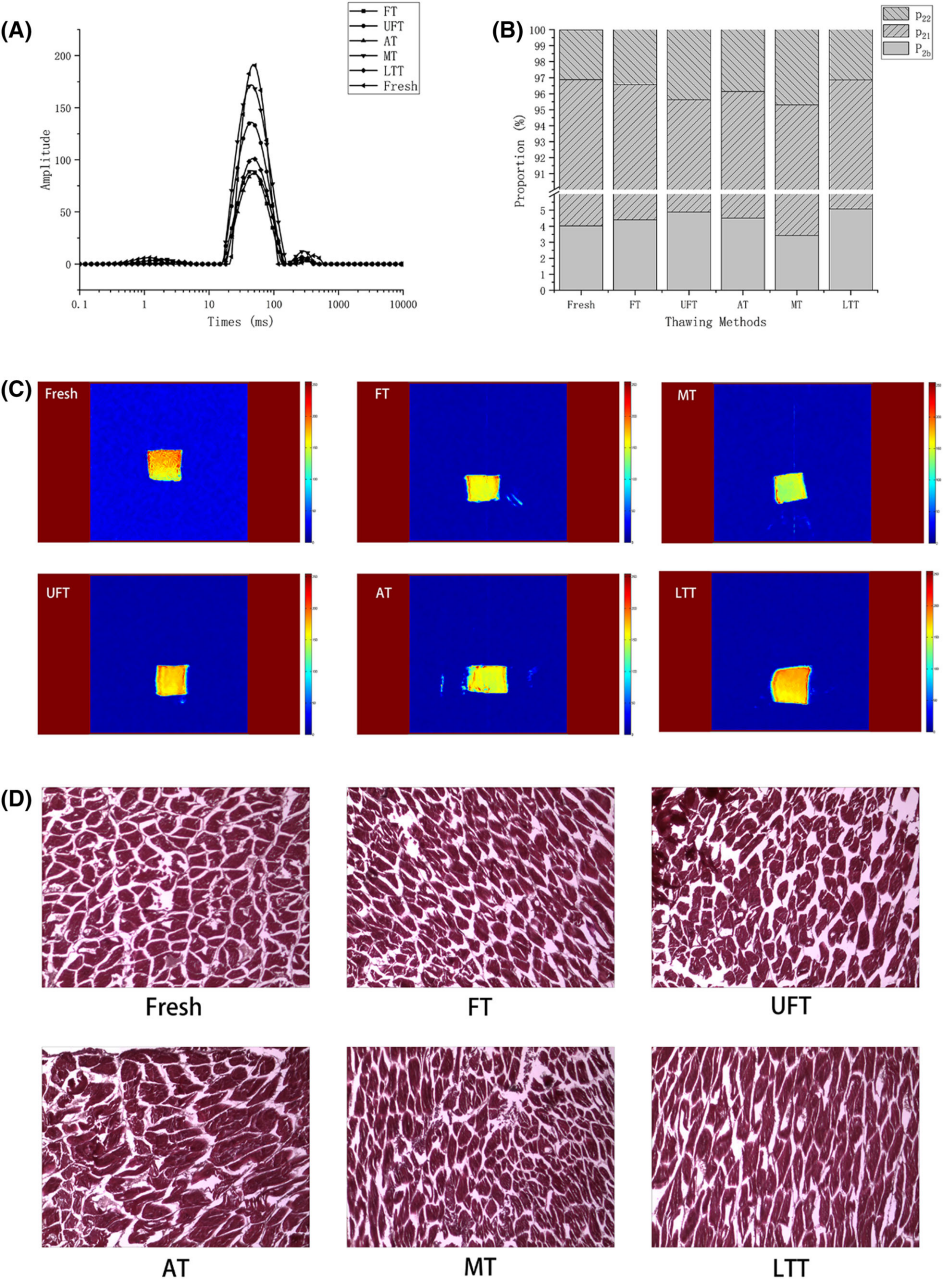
Effects of different thawing methods on the transverse relaxation time (A), distribution of water (B), magnetic resonance image (C) and microstructure (× 40) of mackerel
